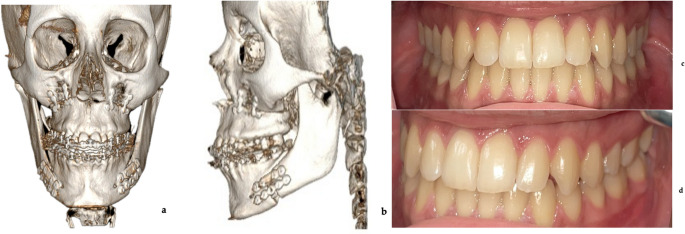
Post-operative images of case 1 (a) post-operative frontal view CT scan (b) post-operative lateral view CT scan (c) final occlusion in frontal view (d) final left lateral occlusion
